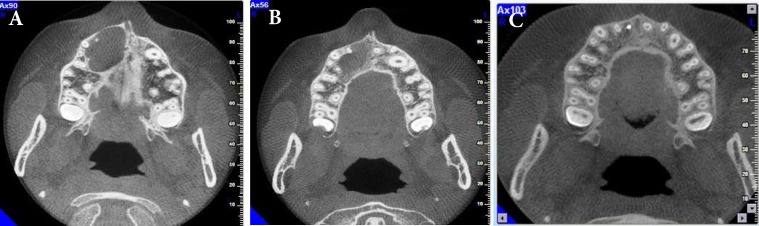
CBCT view; *A)* first visit; *B)* after 6 months, Significant shrinkage in the size of the lesion was observed; *C)* After 20 months of follow-up
